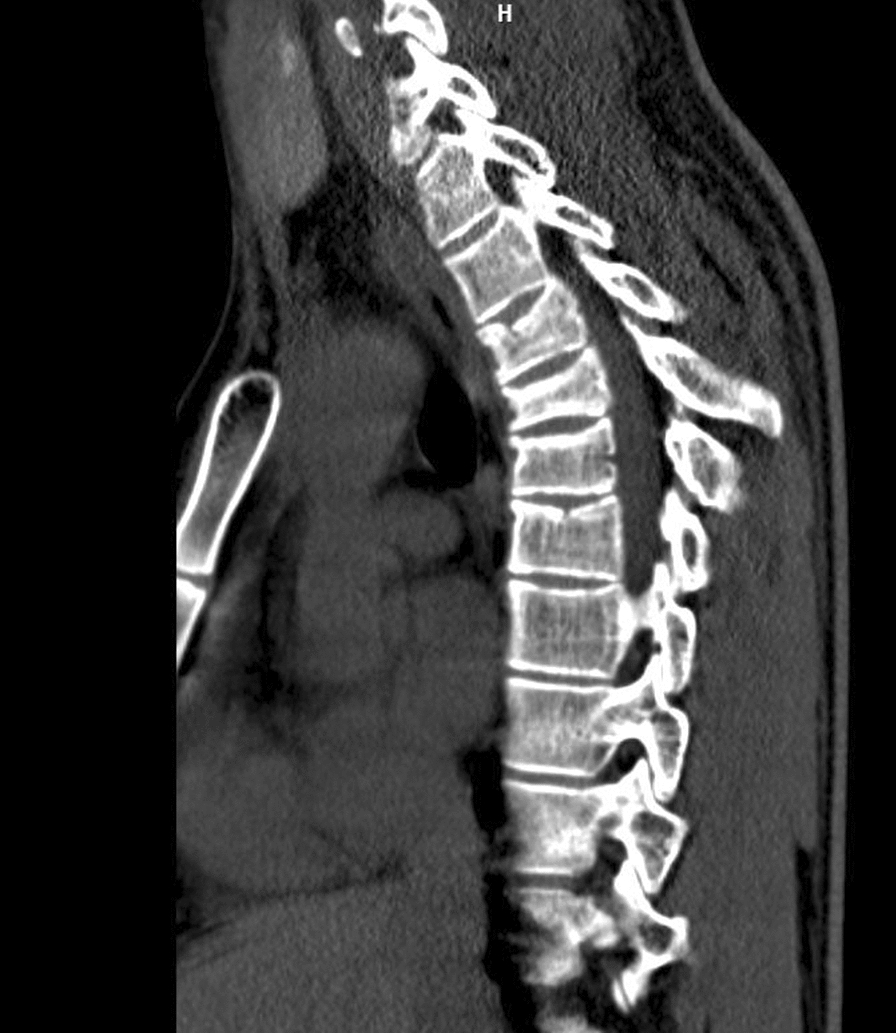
CT scan showing multiple compression fractures of thoracic vertebrae
